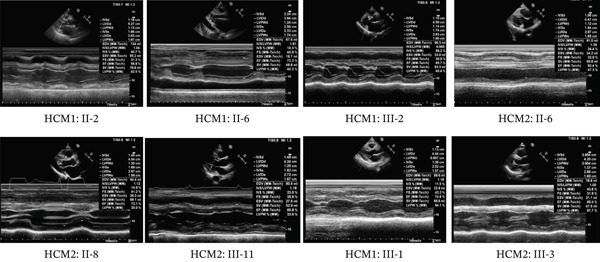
Clinical examination of matrilineal relatives who suffered from HCM using a two‐dimensional echocardiogram.
